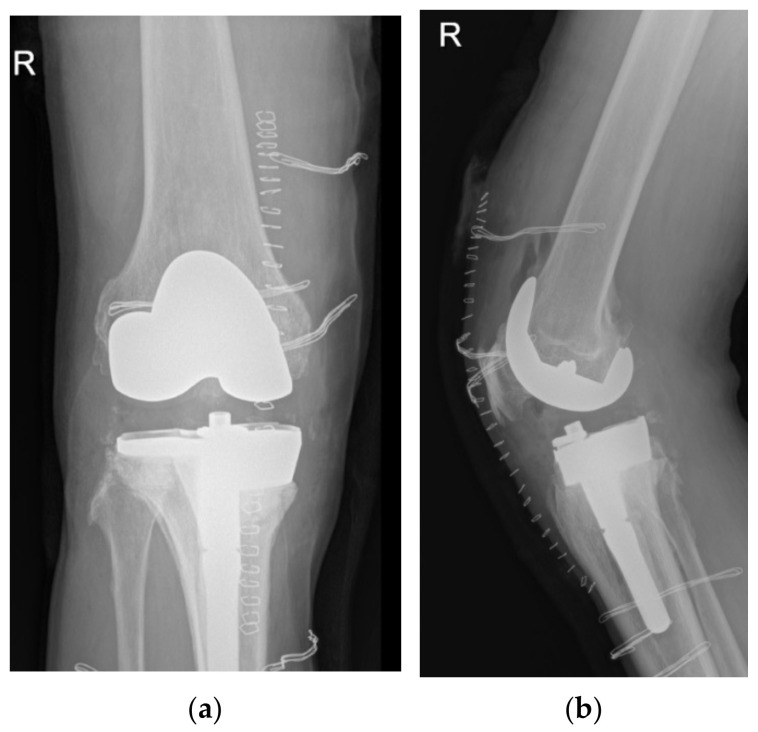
Anteroposterior (a) and lateral (b) postoperative right knee radiographs depicting the revision total knee construct.
